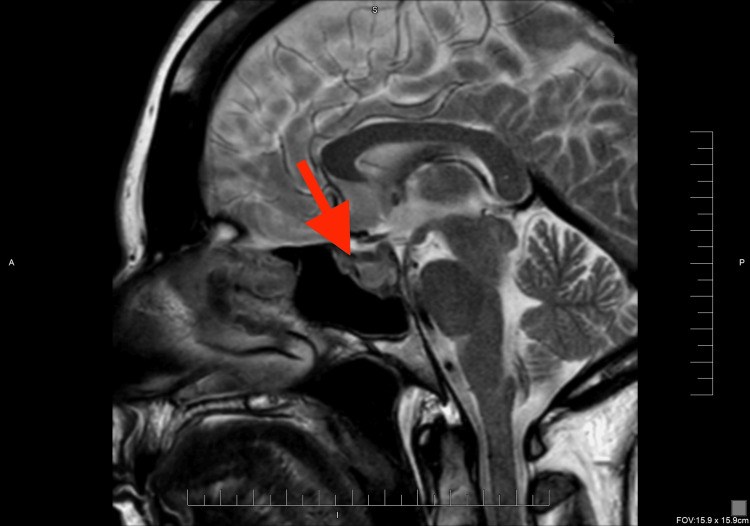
Sagittal T2-weighted MRI On T2 imaging, the same lesion appears heterogeneously hyperintense (red arrow), outlining its suprasellar extension and dural attachment—features compatible with meningioma rather than pituitary macroadenoma.
